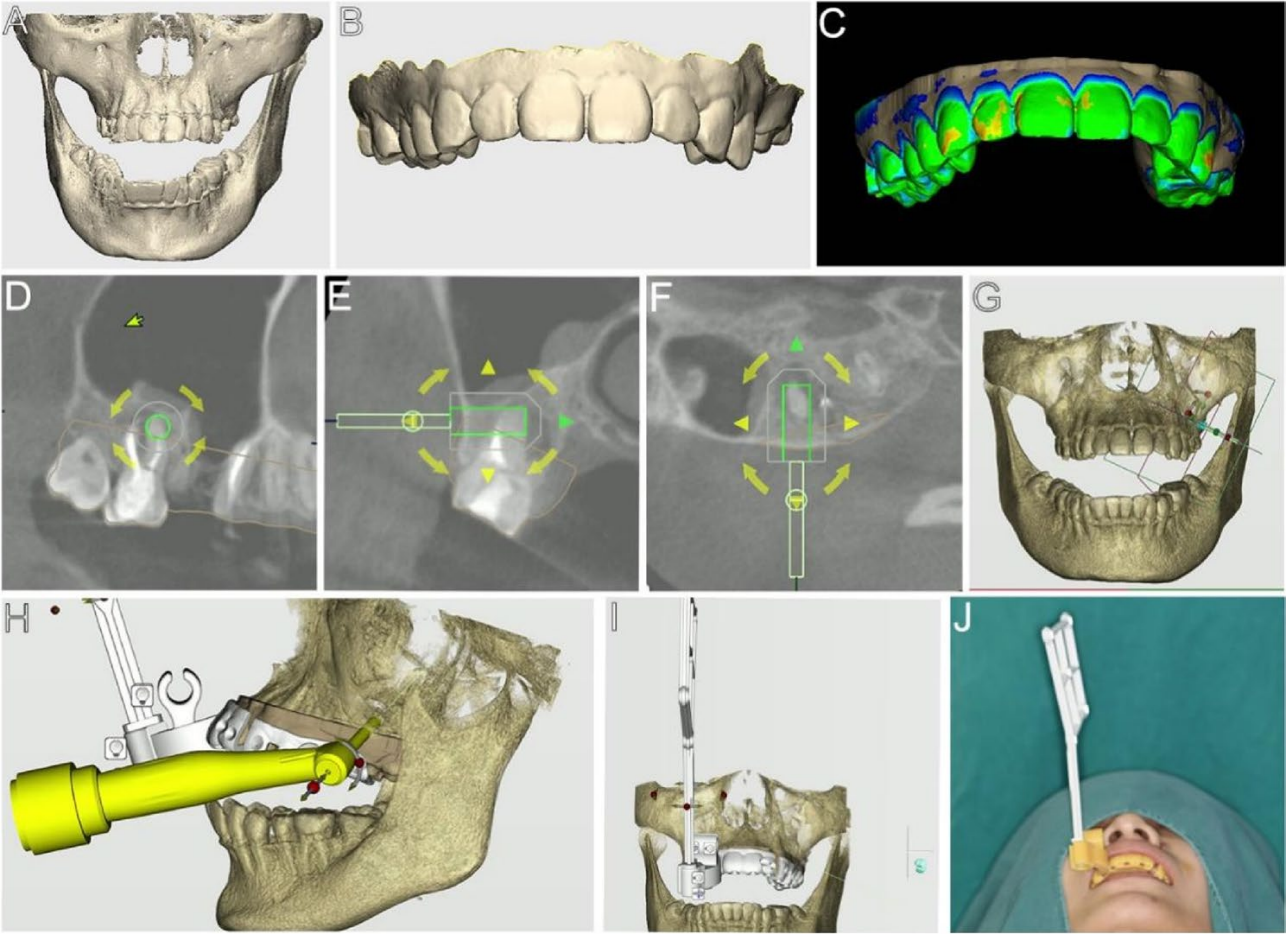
Preoperative preparation. A Jaw data obtained by a CBCT scan. B Intraoral scan data including teeth and soft tissues. C Fit CBCT and intraoral scan data to reconstruct the 3D model. D-H Design drilling path. I-J Design and manufacture surgical personalized accessories and visual marker components
